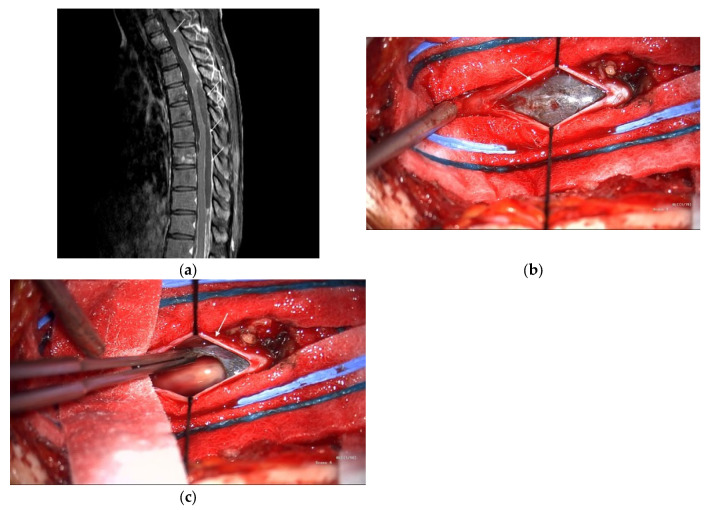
(a) Spine magnetic resonance imaging (MRI) with contrast shows increased enhancement (arrows) along the dural sac of the thoracolumbar spine with several nodular enhancements and increased enhancement of the nerve roots of the lumbar spine. (b) Intraoperative photography showed subdural tumors (arrow). (c) Intraoperative photography showed tumor excision.
